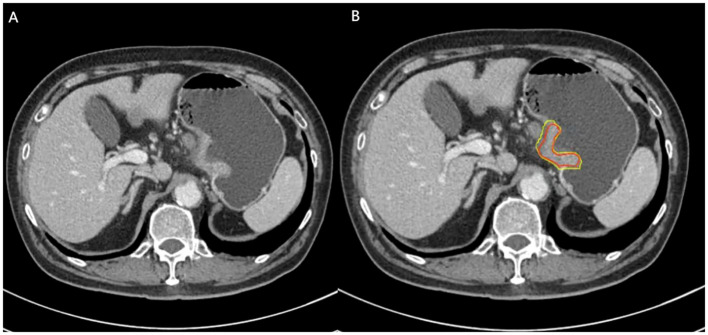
Examples of manual region-of-interest delineation on a portal venous phase CT slice. The intratumoral region (IR) was delineated as VOI1, and the intratumoral plus 3-mm peritumoral region (IPR) was delineated as VOI2 after expansion and manual correction to exclude adjacent bowel, vessels, and organs.
